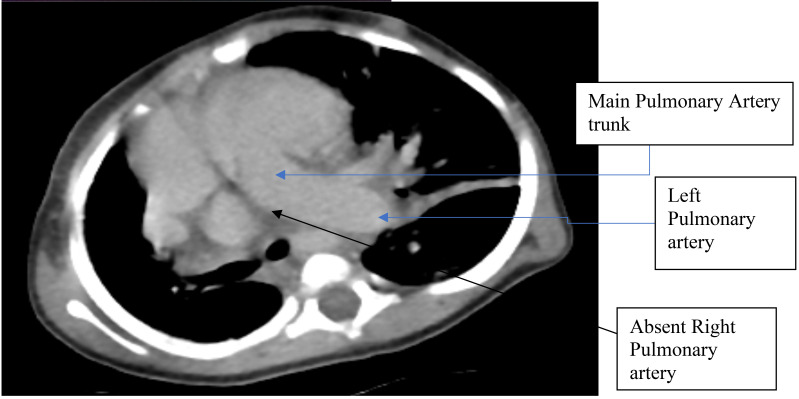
Chest CT-scan displaying absent right pulmonary artery
